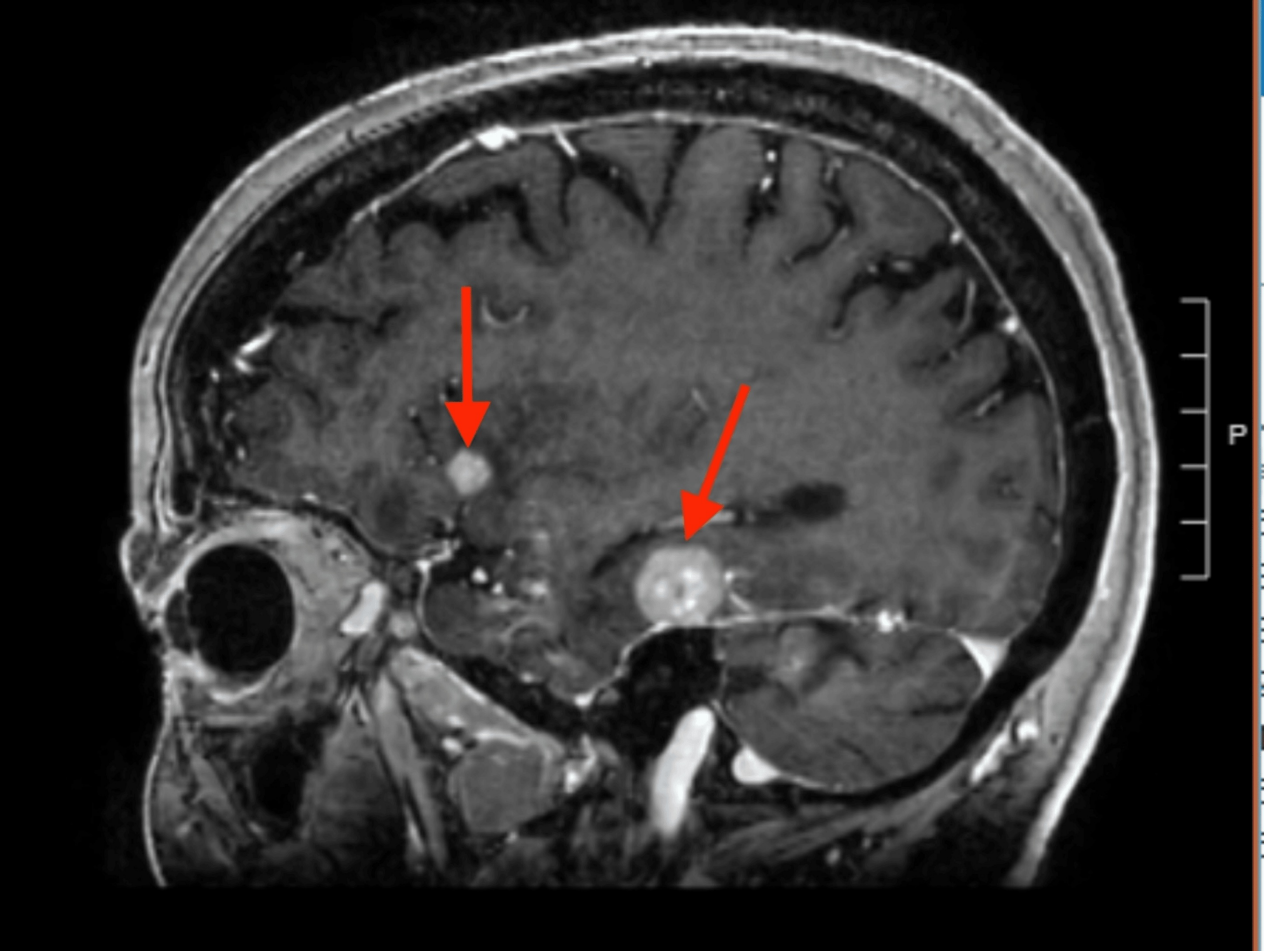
MRI Brain with contrast shows multiple metastatic lesions (arrows)
